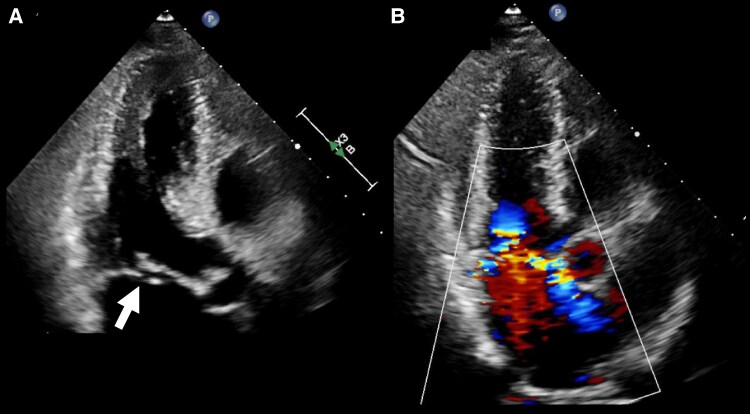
Two-dimensional transthoracic echocardiography of a 73-year-old man. Transthoracic echocardiography revealed prolapse of the posterior mitral leaflet with mobile filamentous structures attached to the leaflet tip (*A*, white arrow), suggesting chordal rupture. Severe eccentric regurgitant jet toward the anterior left atrial wall was observed (*B*). LA, left atrial.
